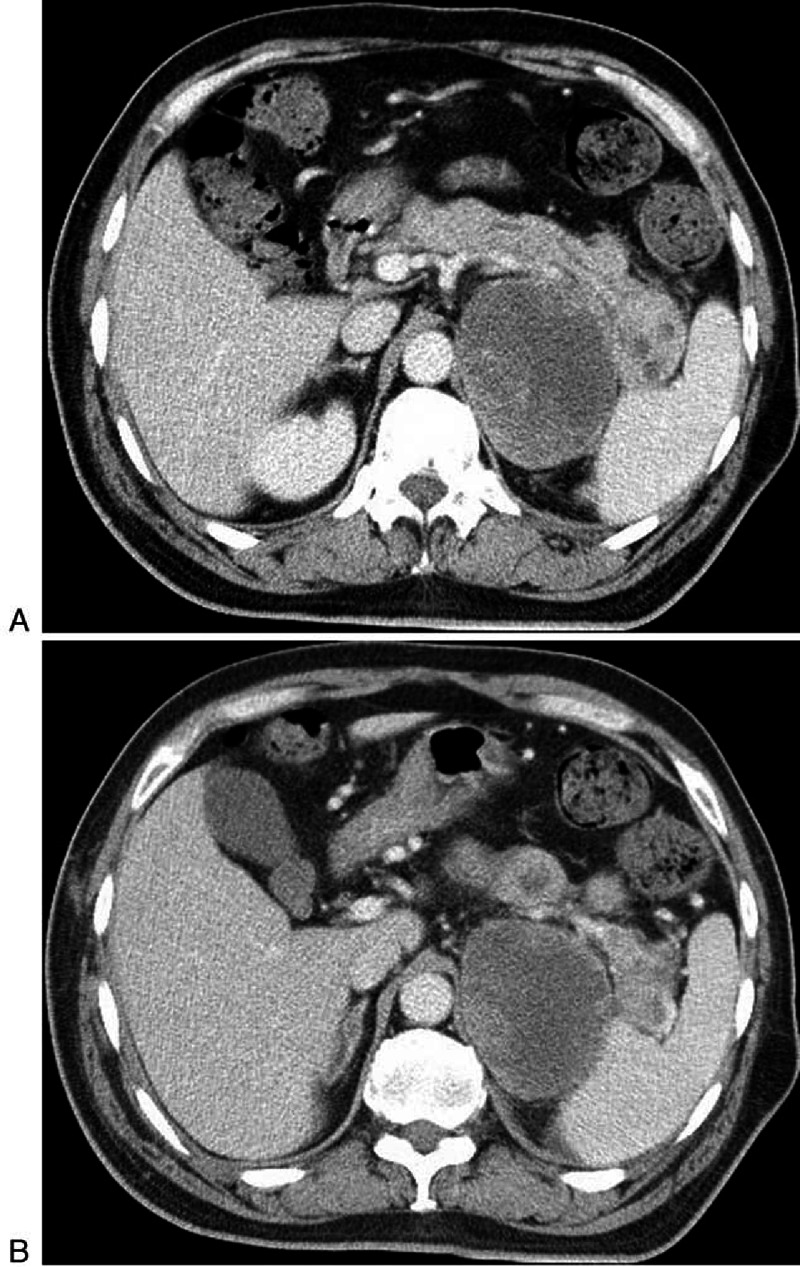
A 58-year-old man with cardiac sarcomas with widespread metastases (patient 16). A-B, Contrast enhanced CT scans show that multiple well-defined and round lesions throughout pancreas with mild dilation of duct. Smaller lesions demonstrate moderate and homogeneous enhancement. Larger lesions show heterogeneous enhancement with internal hypodense areas consistent with necrosis.
